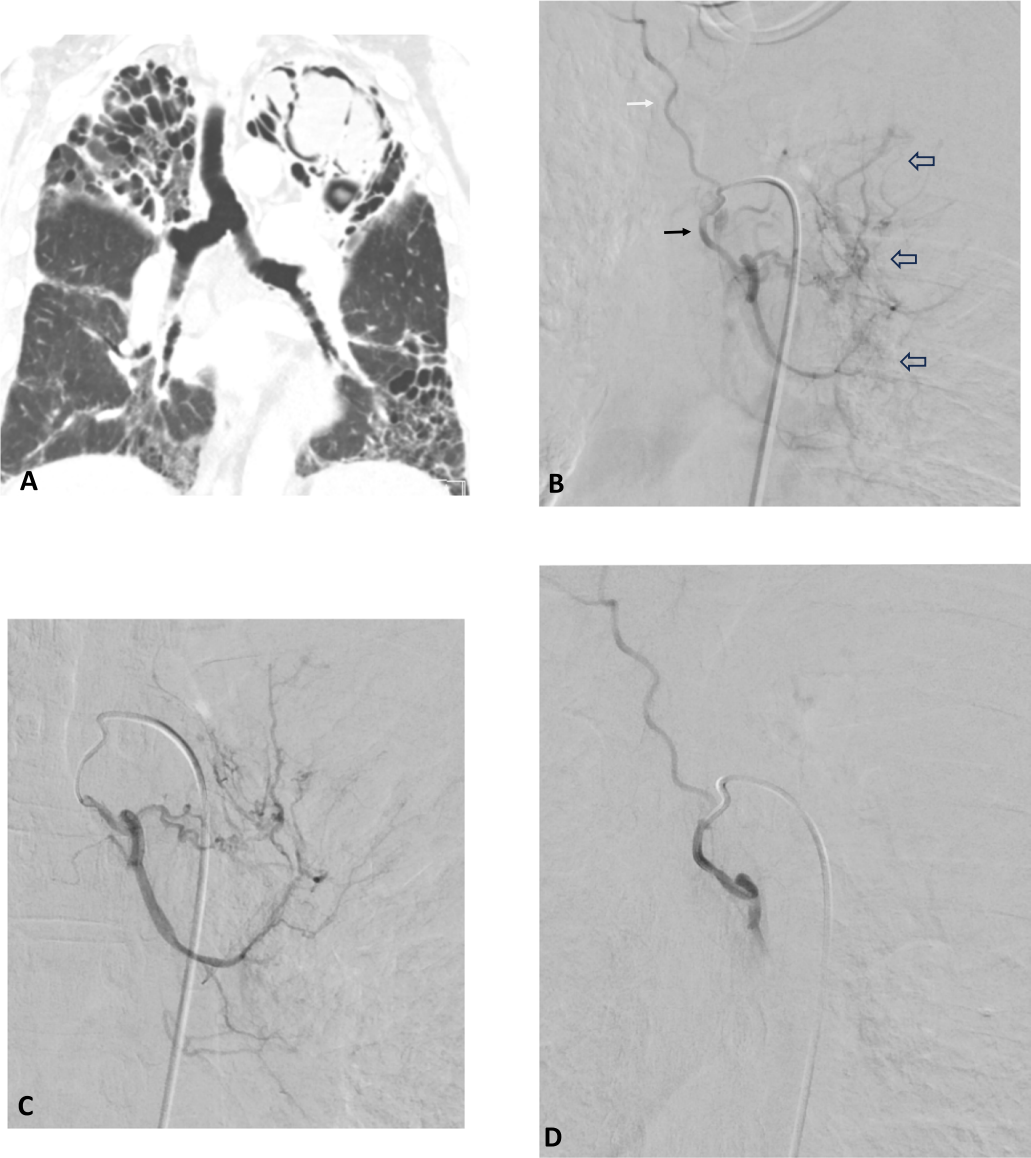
63-year-old man with stage IV sarcoidosis presenting with recurrent haemoptysis. A Coronal CT image shows bilateral upper lobe fibrosis and a large left apical aspergilloma. B Selective left bronchial angiogram of common trunk of R intercostal (white arrow) and L bronchial artery (black arrow). A leash of abnormal branches (open arrows) arising from an enlarged L bronchial artery. C Super-selective L bronchial angiogram prior to embolisation with ns-PVA. D) Completion angiograms shows distal pruning of L bronchial branches and preserved opacification R intercostal artery
